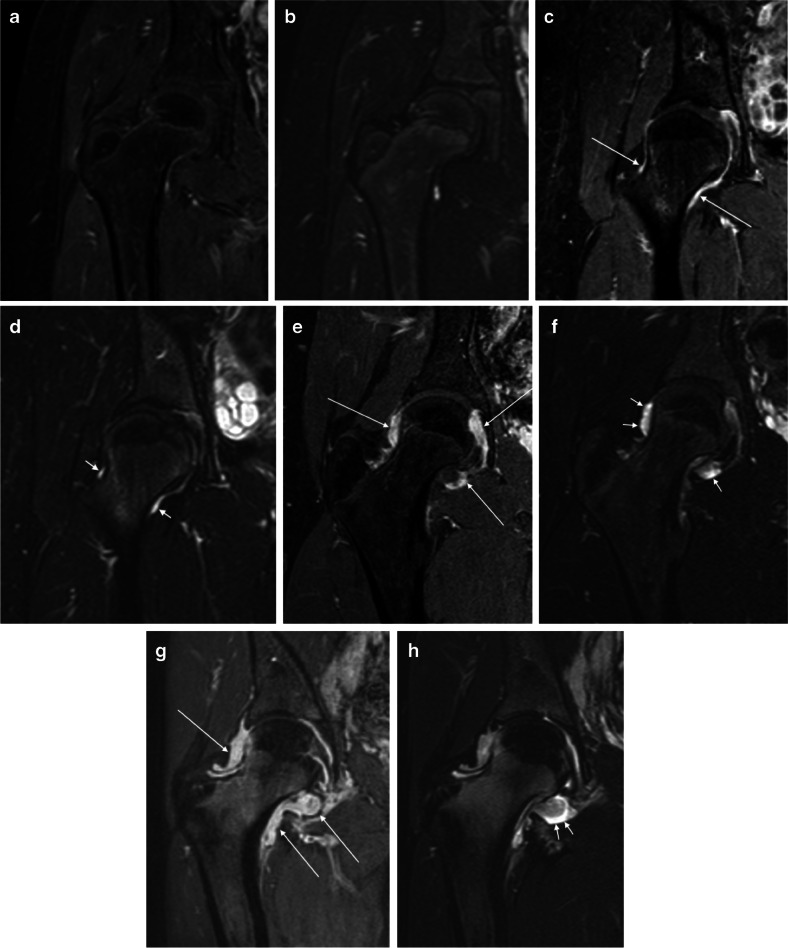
Overall degree of inflammation in right-hip MRIs in four pairs of images from four children with juvenile idiopathic arthritis (JIA) across different levels of severity. All images are demonstrated using coronal post-contrast three-dimensional (3-D) gradient echo with fat saturation (a, c, e, g) and coronal fat-saturated T2-weighted turbo spin-echo (b, d, f, h) MRI sequences. a, b No inflammation (score 0) in a 12-year-old boy. c, d Mild synovial thickening with moderate increase in post-contrast enhancement (*long arrows*) and sliver of effusion (*short arrows*) (score 1) in a 16-year-old girl. e, f Moderate synovial thickening with moderately increased post-contrast enhancement (*long arrows*) and mild effusion (*short arrows*) (score 2) in a 15-year-old boy. g, h Severe synovial thickening and increased post-contrast enhancement, more evident at the medial aspect of the joint (*long arrows*), with mild/moderate effusion (*short arrows*) (score 3) in a 17-year-old girl
